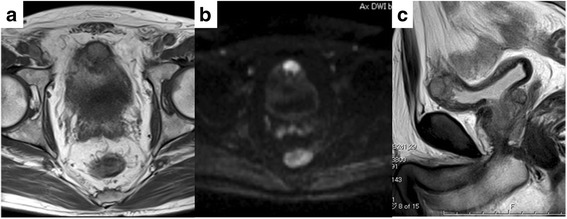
Magnetic resonance imaging in a axial T1-weighted imaging, b axial diffusion, and c sagittal T2-weighted imaging
